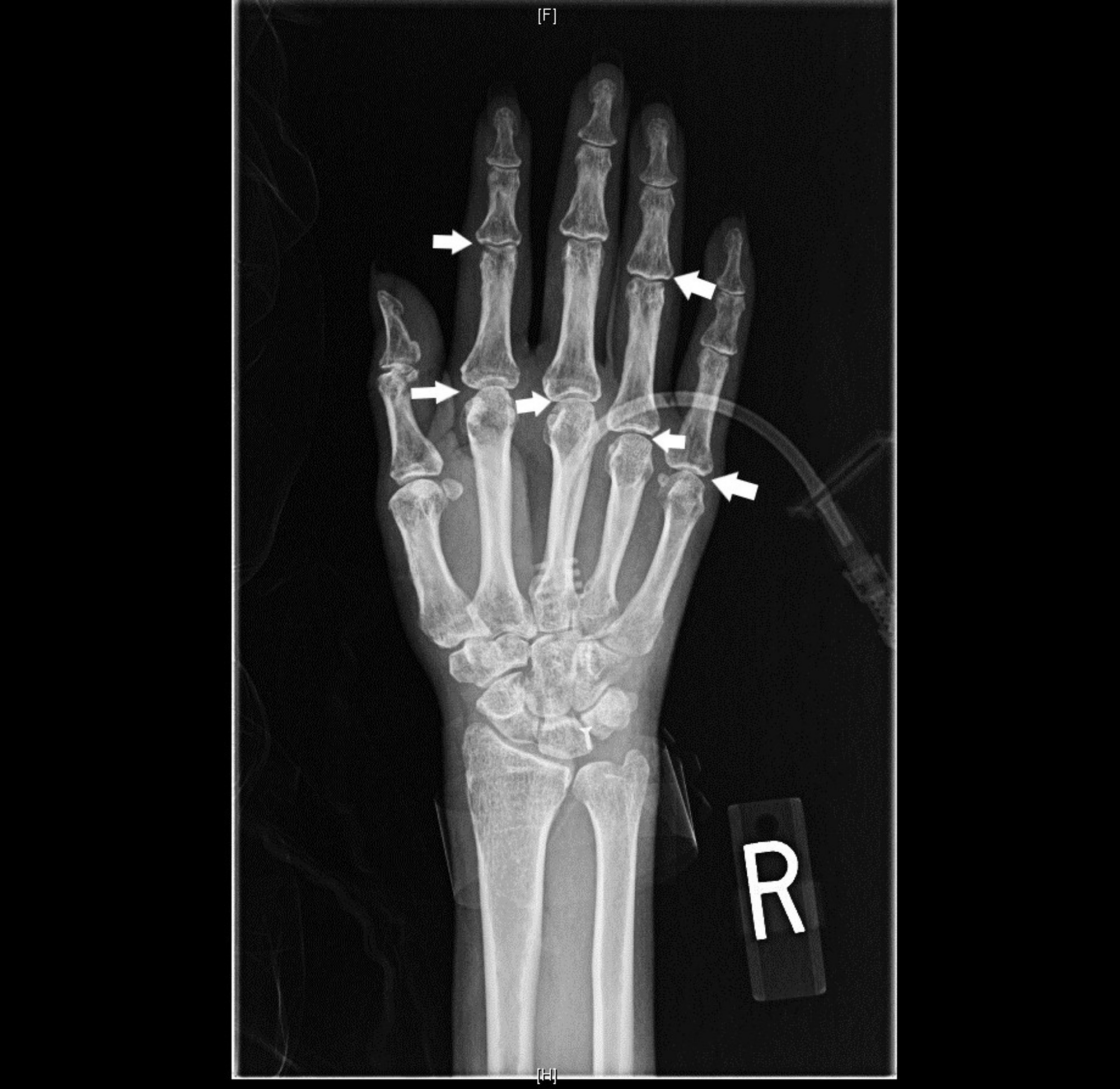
X-ray of the hand showing juxta-articular osteopenia (marked by arrows)
